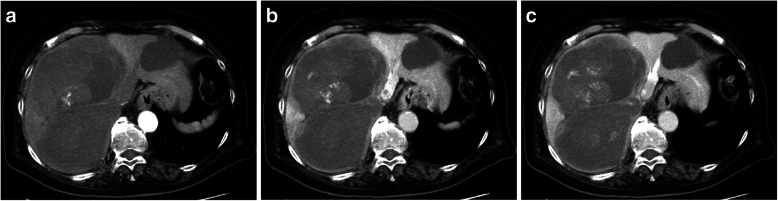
Computed tomography images. A contrast-enhanced dynamic computed tomography image of a tumor 18 cm in diameter in the right hepatic lobe. a Arterial phase, b portal vein phase, and c equilibrium phase
